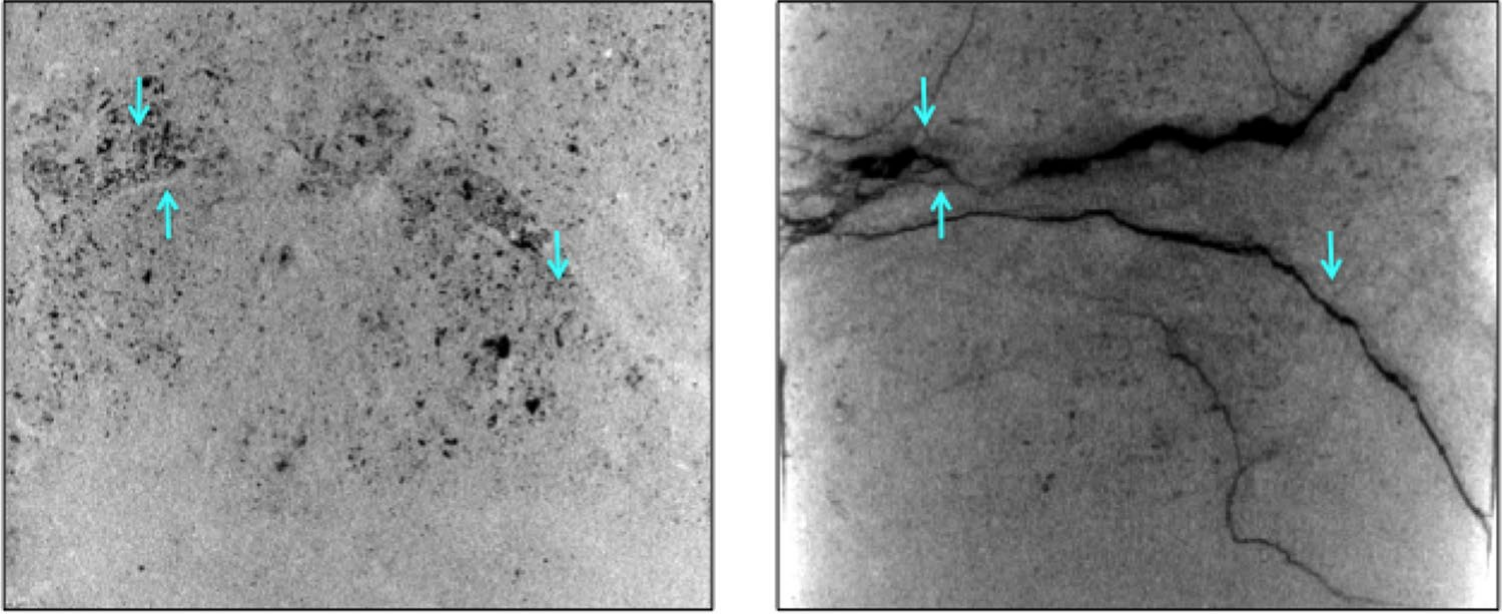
2D vertical slices along the central axis of 3D CT-scans of the third carbonate sample pre- (left) and post-testing (right). Arrows are used to point to the density and, as a result, elastic property contrasts in the pre-test image that developed into fractures clearly visible in the post-test image.
